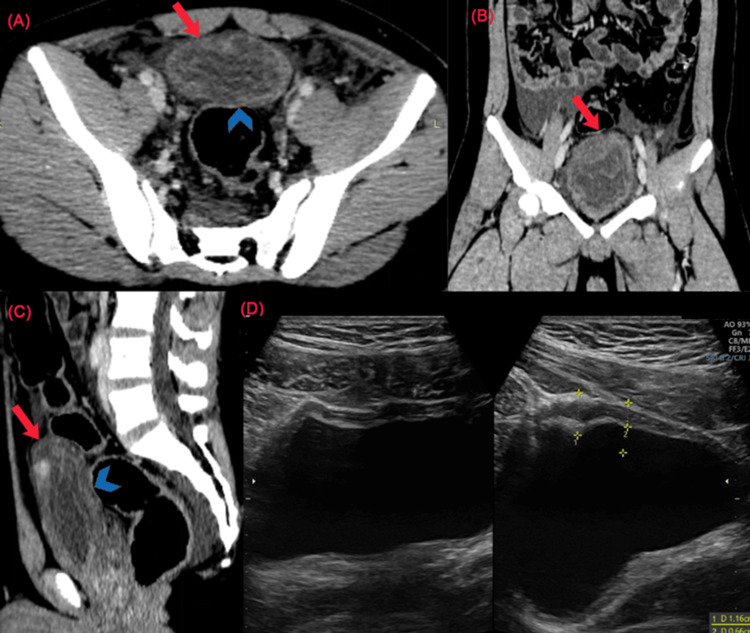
(A-C) Axial, coronal, and sagittal sections of contrast-enhanced CT abdomen and pelvis. (A) Axial section showing diffusely edematous thickening of the urinary bladder wall, predominantly in the superior and posterior aspects (blue arrow). (B) Coronal section showing a focal partial defect (red arrow) in the anterior superior bladder wall. (C) Sagittal section showing diffusely edematous thickening of the superior and posterior bladder wall (blue arrowhead) with a focal partial defect (red arrow) in the anterior superior bladder wall. (D) Follow-up ultrasonography of the abdomen and pelvis showing homogeneous focal thickening of the anterior superior bladder wall without any obvious defect, suggesting resolution in progress.
